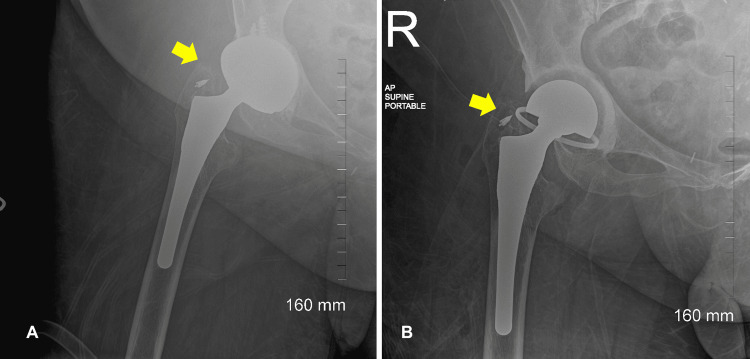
Right hip X-ray before (A) and after (B) debridement and revision
